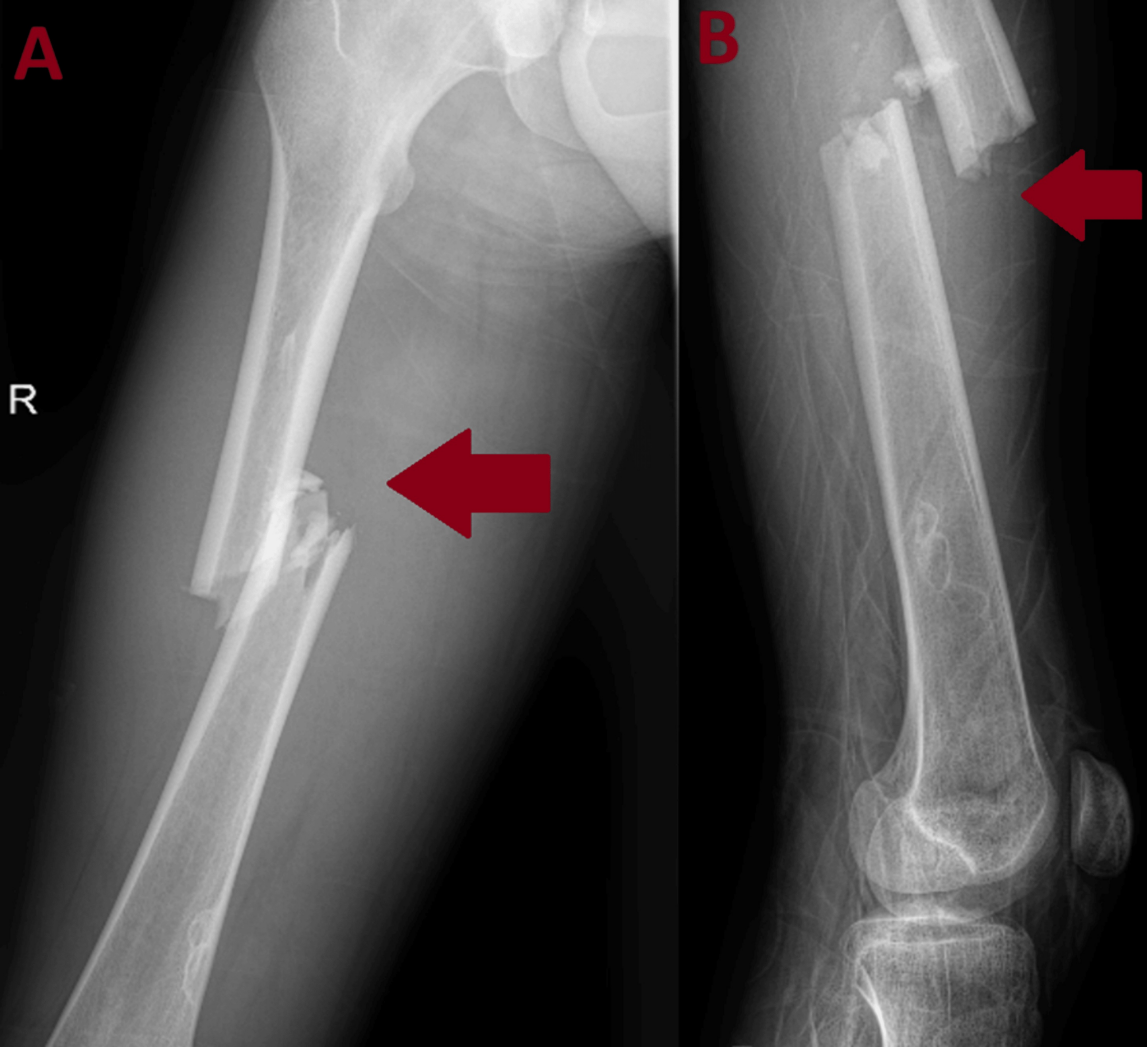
Preoperative images of the right femoral shaft fracture A) Anteroposterior X-ray of the femur. B) Lateral X-ray of the femur
